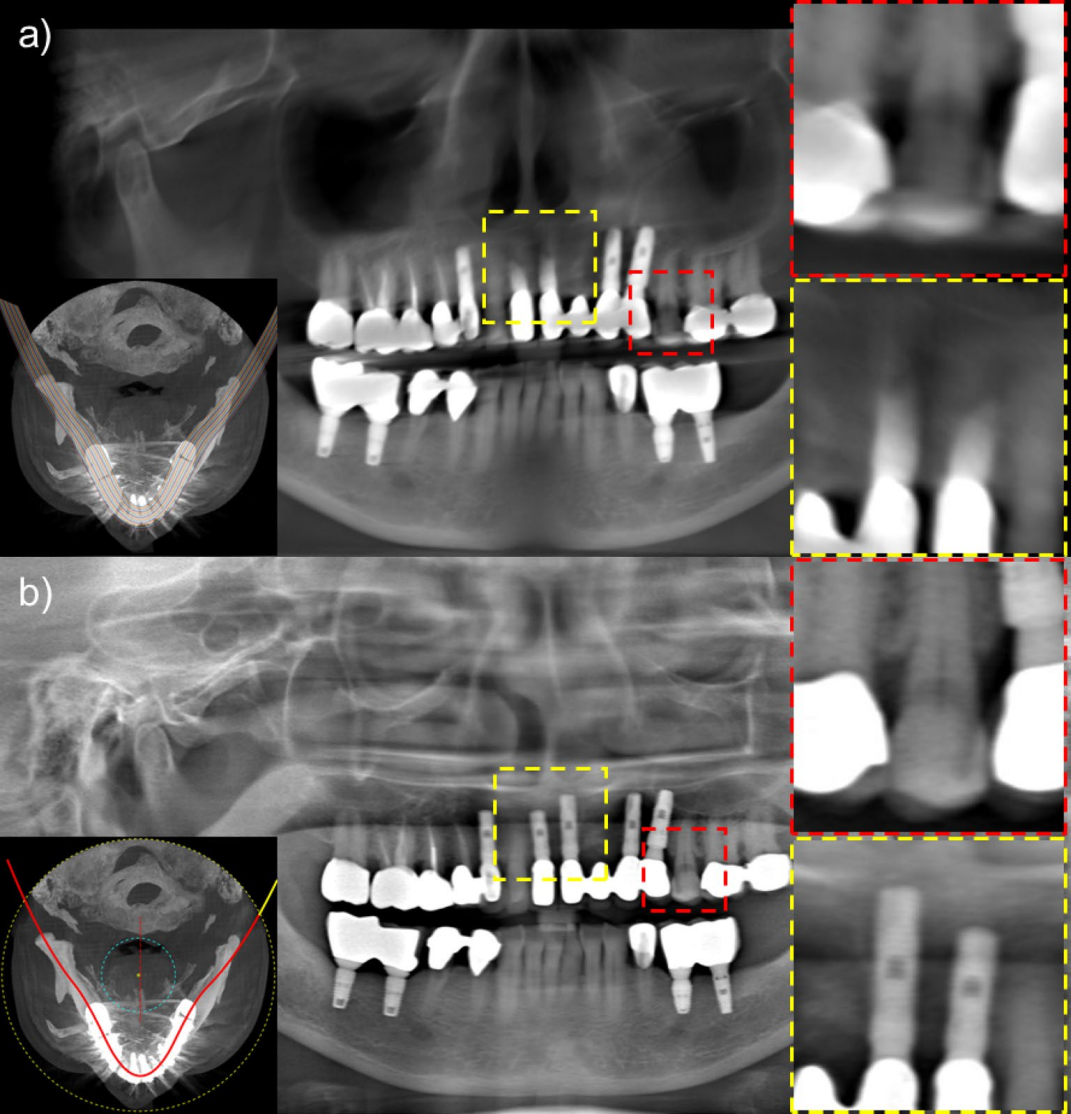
(a) A panoramic image synthesized from the dental CBCT image by use of a conventional method, (b) A panoramic image obtained by the proposed method.
